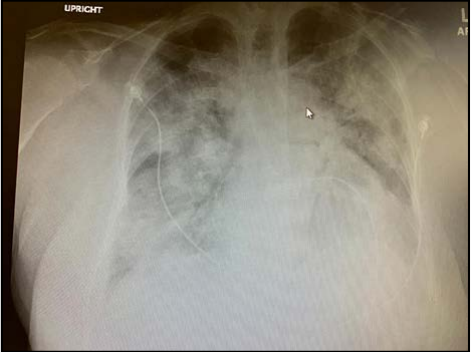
Chest x-ray of a PCR-positive adult with severe COVID-19 Pneumonia, showing extensive bilateral peripheral ground glass haze- (arrow), and consolidation (Image courtesy: Dr Imran Sharief, MD, FCCP)
